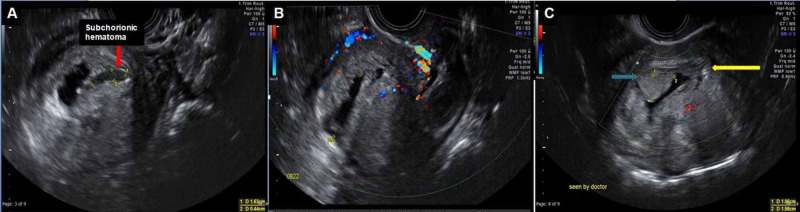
Transvaginal ultrasound during the first trimester (A-C) A distorted low-lying intrauterine gestation sac (yellow arrow) is seen, measuring 28 mm. A subchorionic heterogeneously hypoechoic lesion (red arrow) with no internal vascularity on color Doppler (B) is consistent with subchorionic hematoma. An oval-shaped protrusion from the chorion (isoechoic to the chorion) into the gestational sac, measuring 1 X 1.9 cm, with no vascularity on color Doppler, is suggestive of the chorionic bump.
